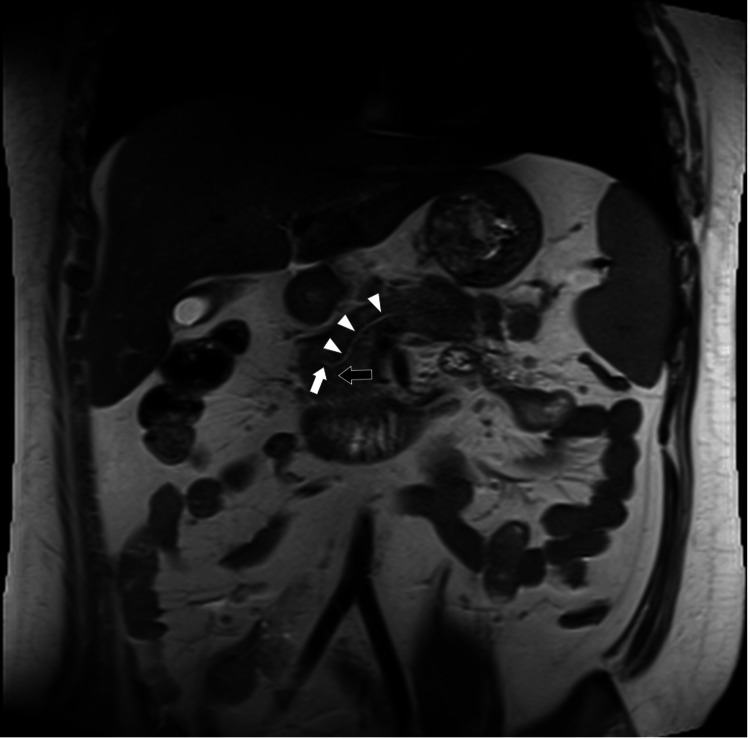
T2-weighted coronal image of incomplete pancreatic divisum showing dorsal pancreatic duct (white arrow) connecting to the ductus Santorini (white arrow heads) and opening into the accessory pancreatic duct. The ventral pancreatic duct (black arrow) is thin and considered an incomplete type of pancreatic divisum.
